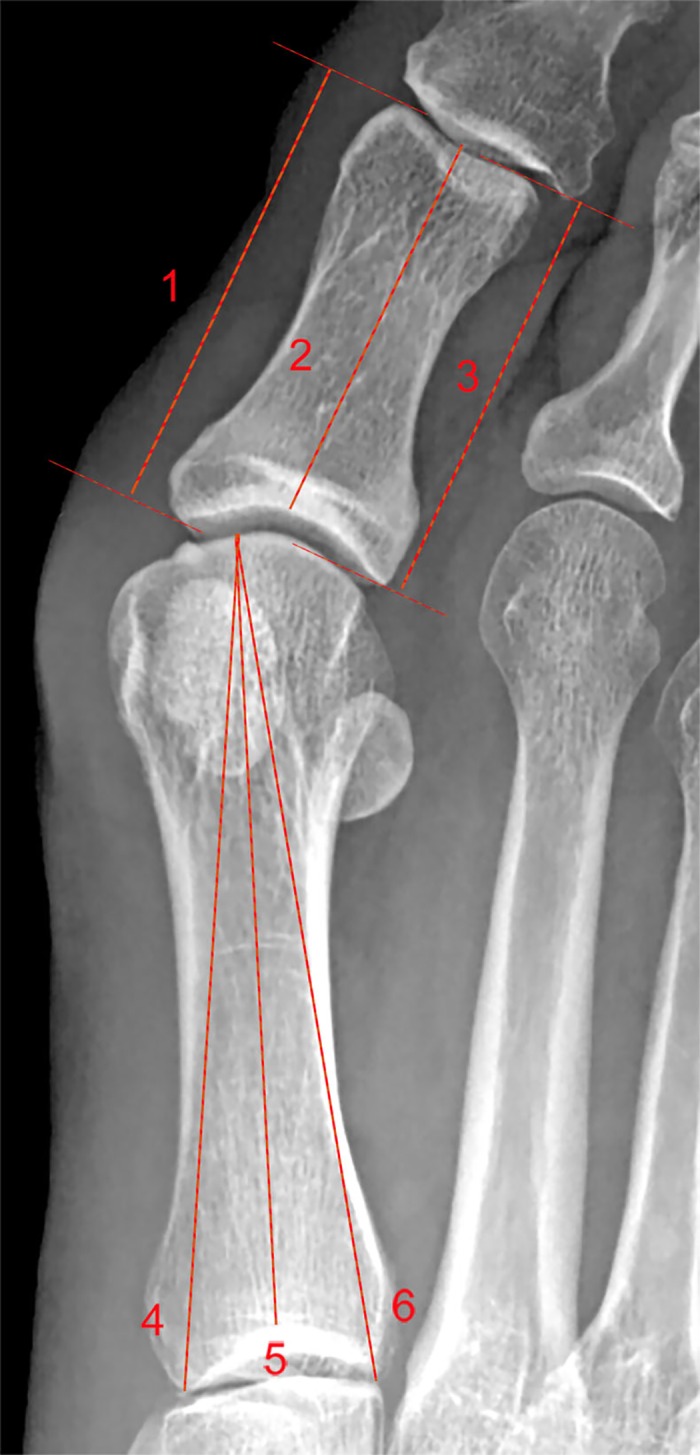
On the weight-bearing dorsoplantar foot radiograph, lines were measured and drawn; line 1 is the longitudinal distance medial aspect of the proximal phalanx (LDMPPH); line 2 is the longitudinal distance central aspect of the proximal phalanx (LDCPPH); line 3 is the longitudinal distance lateral aspect of the proximal phalanx (LDLPPH); line 4 is the longitudinal distance medial aspect of the I metatarsal bone (LDM-M); line 5 is the longitudinal distance central aspect of the I metatarsal bone (LDC-M); line 6 is the longitudinal distance lateral aspect of the I metatarsal bone (LDL-M).
